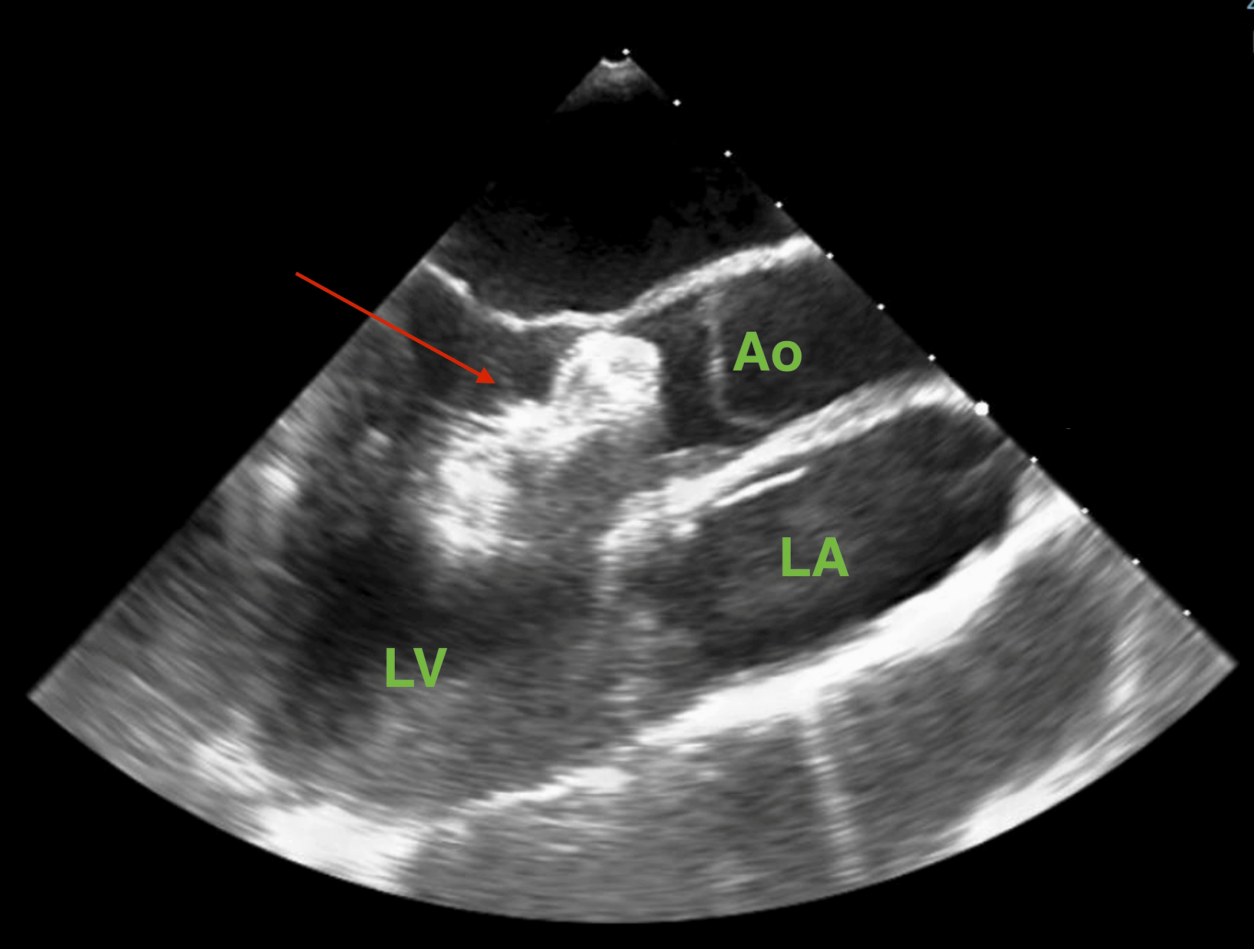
Echocardiography showing the atrial septal occluder trapped in the left ventricular outflow tract. Ao: aorta; LA: left atrium; LV: left ventricle Red arrow: Amplatzer Septal Occluder This echocardiography was performed seven weeks after atrial septal occluder implantation.
